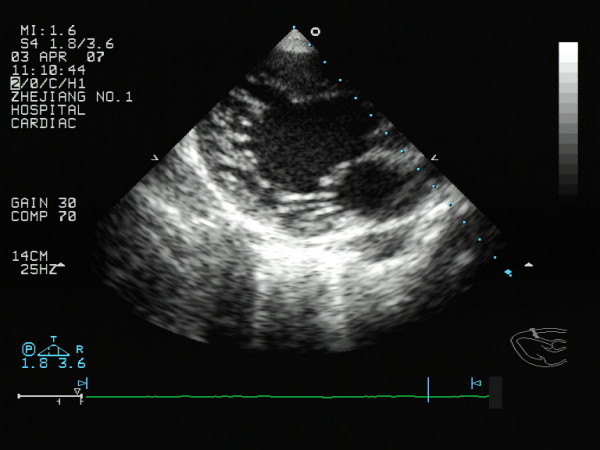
left ventricular long axis view showing the trabeculationsof the left ventricular wall and deep intertrabecular recesses (arrow) by two-dimension echocardiograms.
